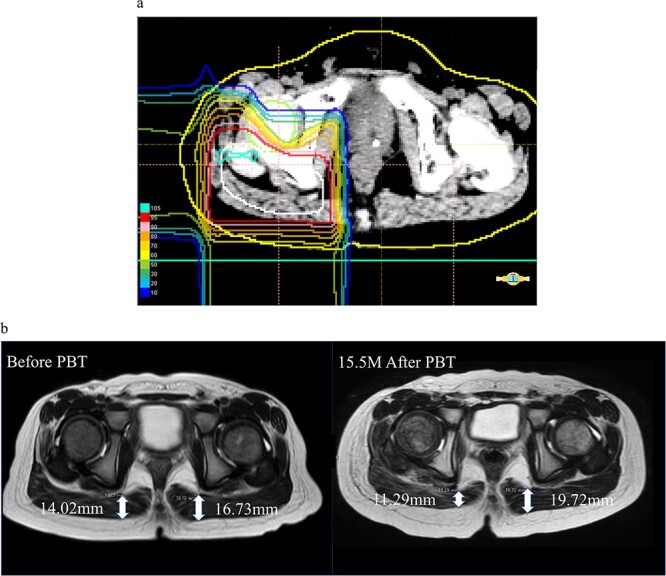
Case of a 5-year-old boy with Ewing’s sarcoma of the right hip. (a) Dose distribution of PBT. (b) Thickness of the irradiated and contralateral side muscles on MRI before PBT (left) and MRI at 15.5 months after PBT (right). The same cross-section is shown in the images before and after PBT.
